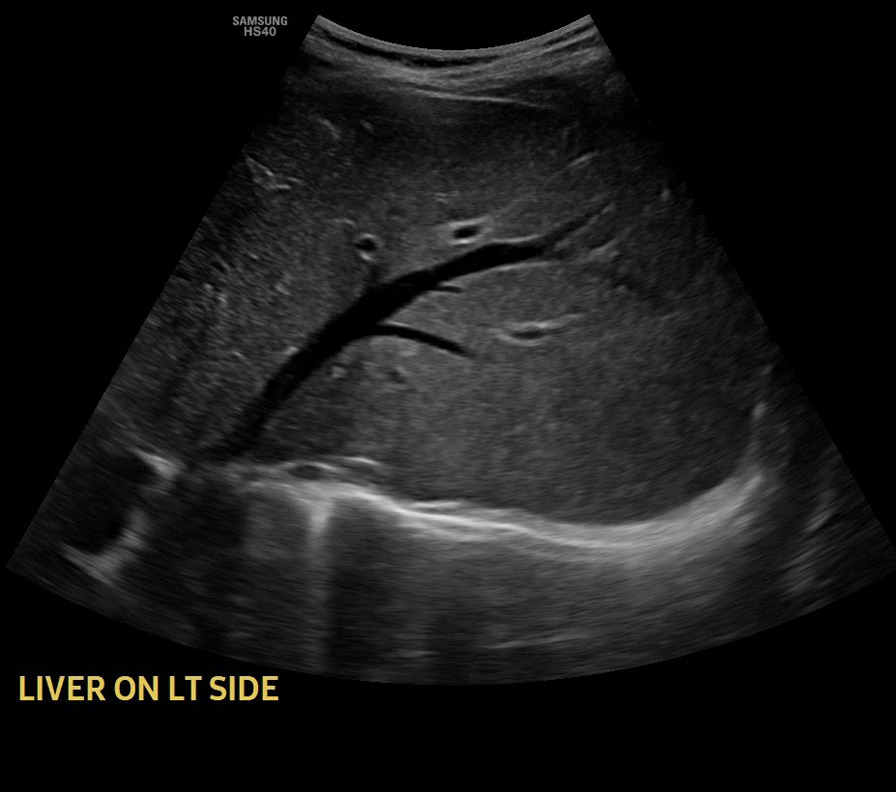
Sonographic picture of normal liver found on the left side
